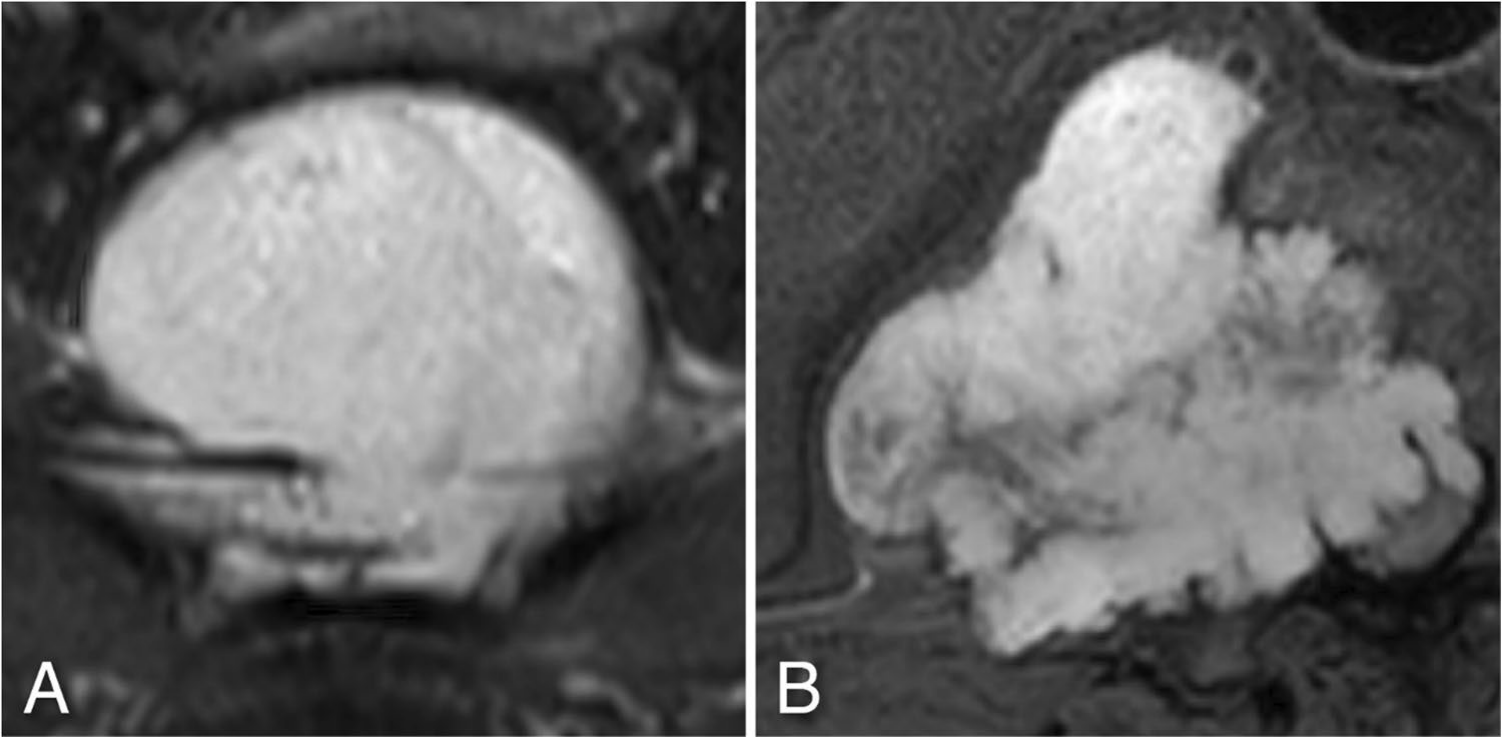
Examples of tumor shape. A- Smooth shape seen in a midline located chordoma of the sacrum with intraspinal-extraspinal extension on T2 fat-saturated MR image. B- Lobulated shape seen in an eccentrically located chondrosarcoma of the mobile spine with intraspinal-extraspinal extension on T2 fat-saturated MR image
